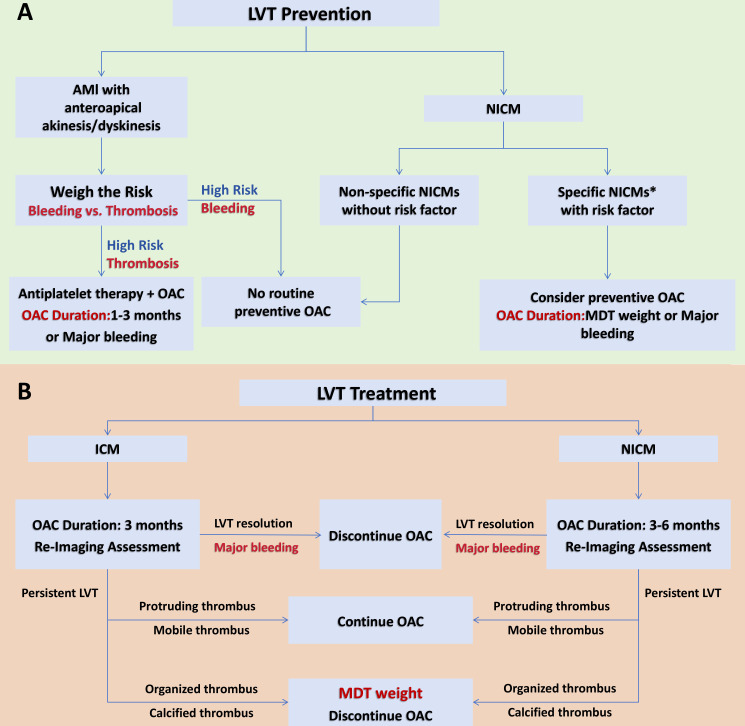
Proposed prevention modalities for LVT. AMI, acute myocardial infarction; ICM, ischaemic cardiomyopathies; LVT, left ventricular thrombus; MDT, multidisciplinary team; NICM, non-ischaemic cardiomyopathies; OAC, oral anticoagulants. *CT was chosen when cardiovascular MR was unavailable or not tolerated.
